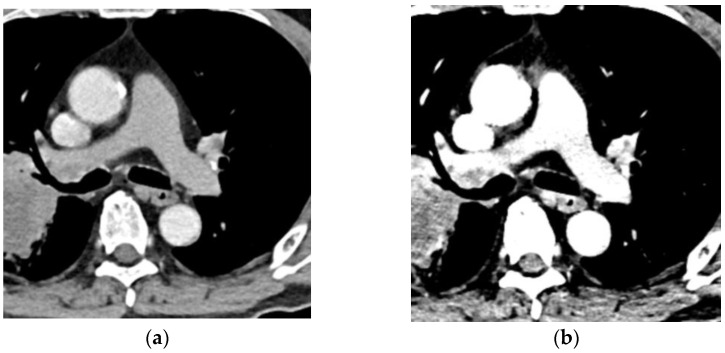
CT pulmonary angiography with a 50% reduction in the contrast medium: (a) Transverse CT pulmonary angiography at 120 kVp; (b) Noise-optimized virtual monochromatic image at 40 keV. The CT numbers in the pulmonary trunk are 95 HU at 120 kVP and 268 HU at 40 keV. The virtual monochromatic image can salvage the insufficient contrast enhancement caused by the reduced volume of contrast medium.
